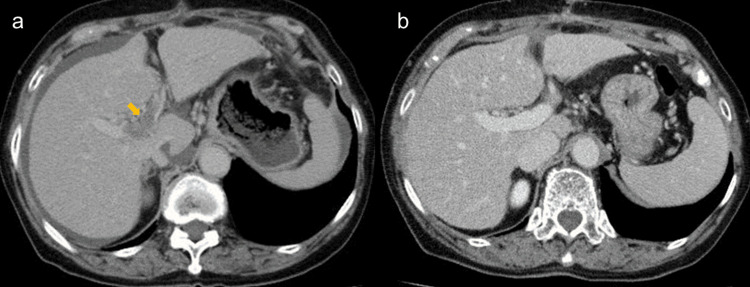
Radiologic findings before and after anticoagulation therapy. (a) Enhanced computed tomography showed portal vein thrombosis (arrow) and ascites on postoperative day 15. (b) Enhanced computed tomography showed complete remission of thrombosis on postoperative day 25.
